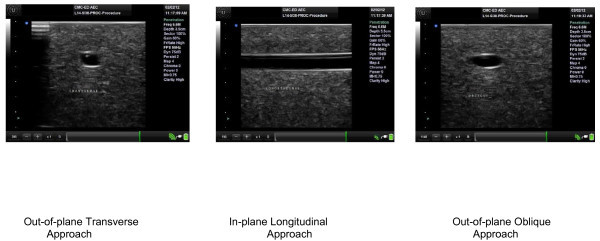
Sonographic view of each approach. Out-of-plane Transverse Approach, In-plane Longitudinal Approach, Out-of-plane Oblique Approach.
